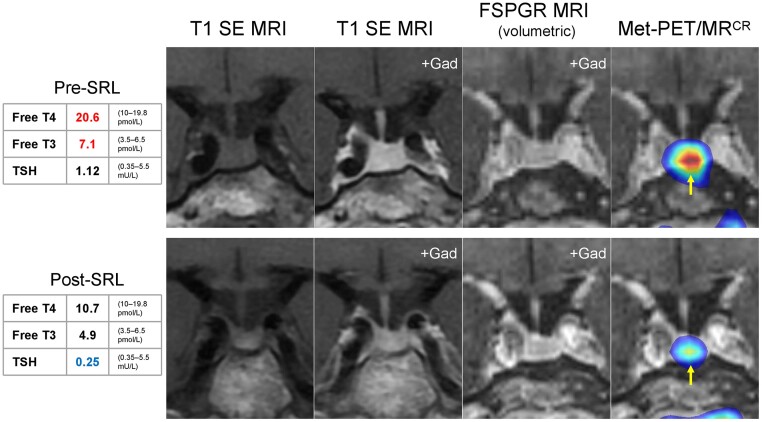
Molecular (functional) pituitary imaging in a patient with an occult microthyrotropinoma. Standard clinical MRI (coronal T1SE before and after gadolinium) at diagnosis fails to demonstrate an adenoma. Similarly, volumetric (FSPGR) sequences are unremarkable. However, molecular imaging with Met-PET coregistered with FSPGR MRI reveals intense focal radiotracer uptake just to the left of the site of insertion of the infundibulum. Following 3 months of depot SRL therapy, with resultant normalization of thyroid function tests, there is no discernible change in anatomical imaging findings; however, in marked contrast, there is dramatic diminution in radiotracer uptake, thereby revealing the location of the occult microadenoma (subsequently confirmed at transsphenoidal surgery). Abbreviations: FSPGR, fast spoiled gradient recalled echo; Gad, gadolinium; Met-PET/MR^CR^, ^11^C-methionine PET/CT coregistered with FSPGR MRI; MRI, magnetic resonance imaging; PET, positron emission tomography; SE, spin echo; SRL, somatostatin receptor ligand; T3, triiodothyronine; T4, thyroxine; TSH, thyroid-stimulating hormone.
